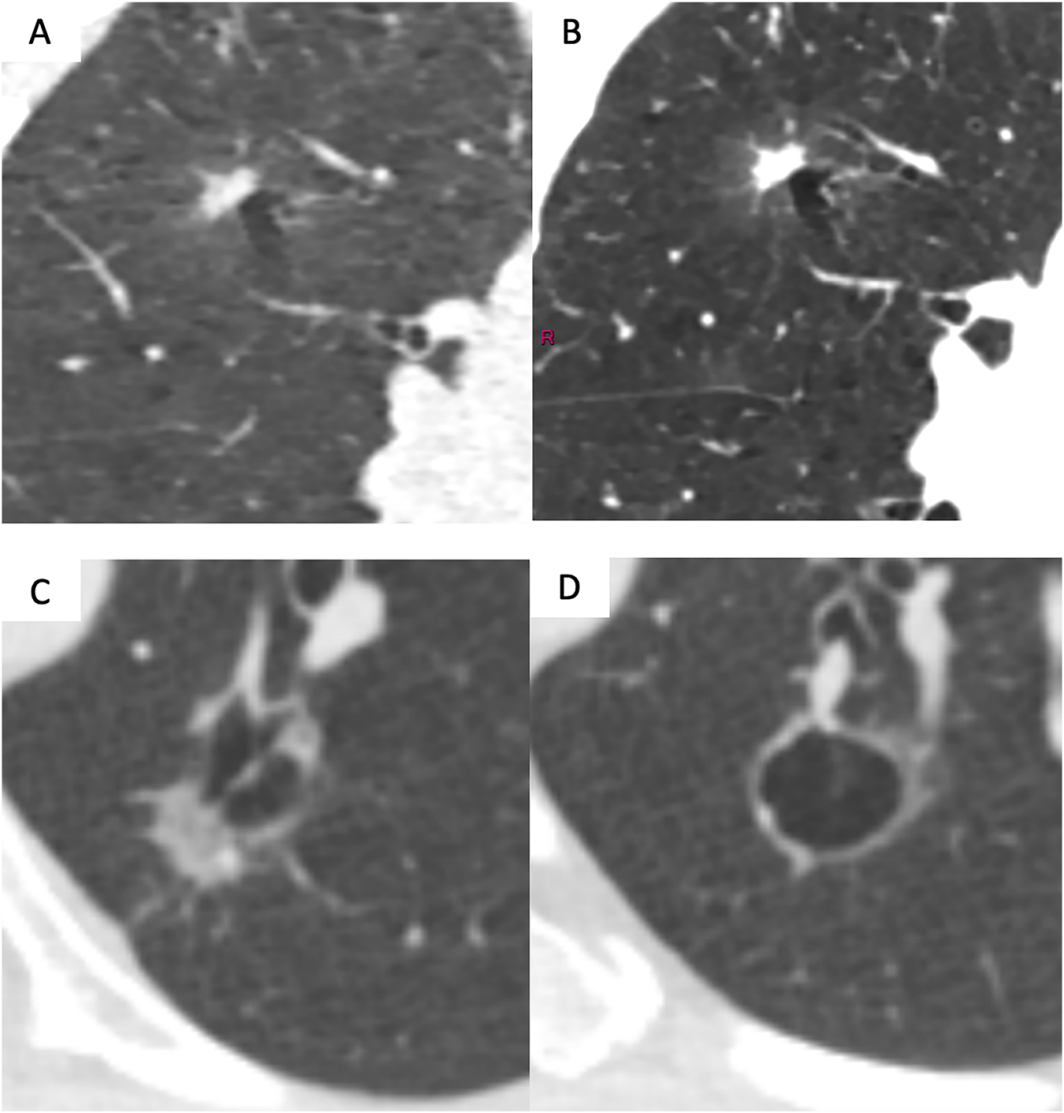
Suspicious morphological sign of ‘atypical cyst’ or ’cystic airspace’ in two patients. A, B Low-dose coronal CT images showing a 7-mm solid nodule in the wall of a right upper lobe cyst in a 60-year-old woman who smoked 31 pack-years, corresponding to an invasive adenocarcinoma staged pT1bN0R0. Standard-dose CT acquisition (B) performed preoperatively revealed a ground-glass component that was not detected on low-dose acquisition (A). C, D Low-dose axial CT images showing an atypical cyst of the left lower lobe in a 62-year-old woman with a smoking history of 40 pack-years. A solid part of 12 mm is extrinsically developed. A pT1b invasive adenocarcinoma was confirmed postoperatively
